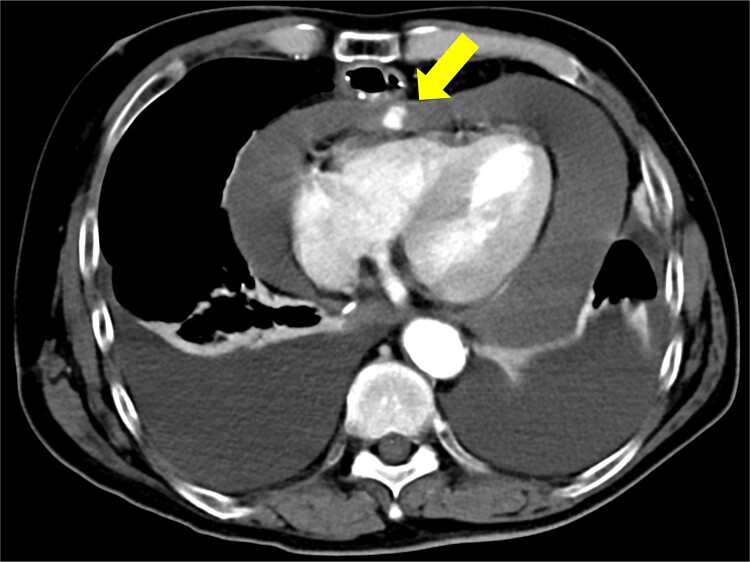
Contrast-enhanced computed tomography shows contrast extravasation in the anterior right ventricle (arrow).
